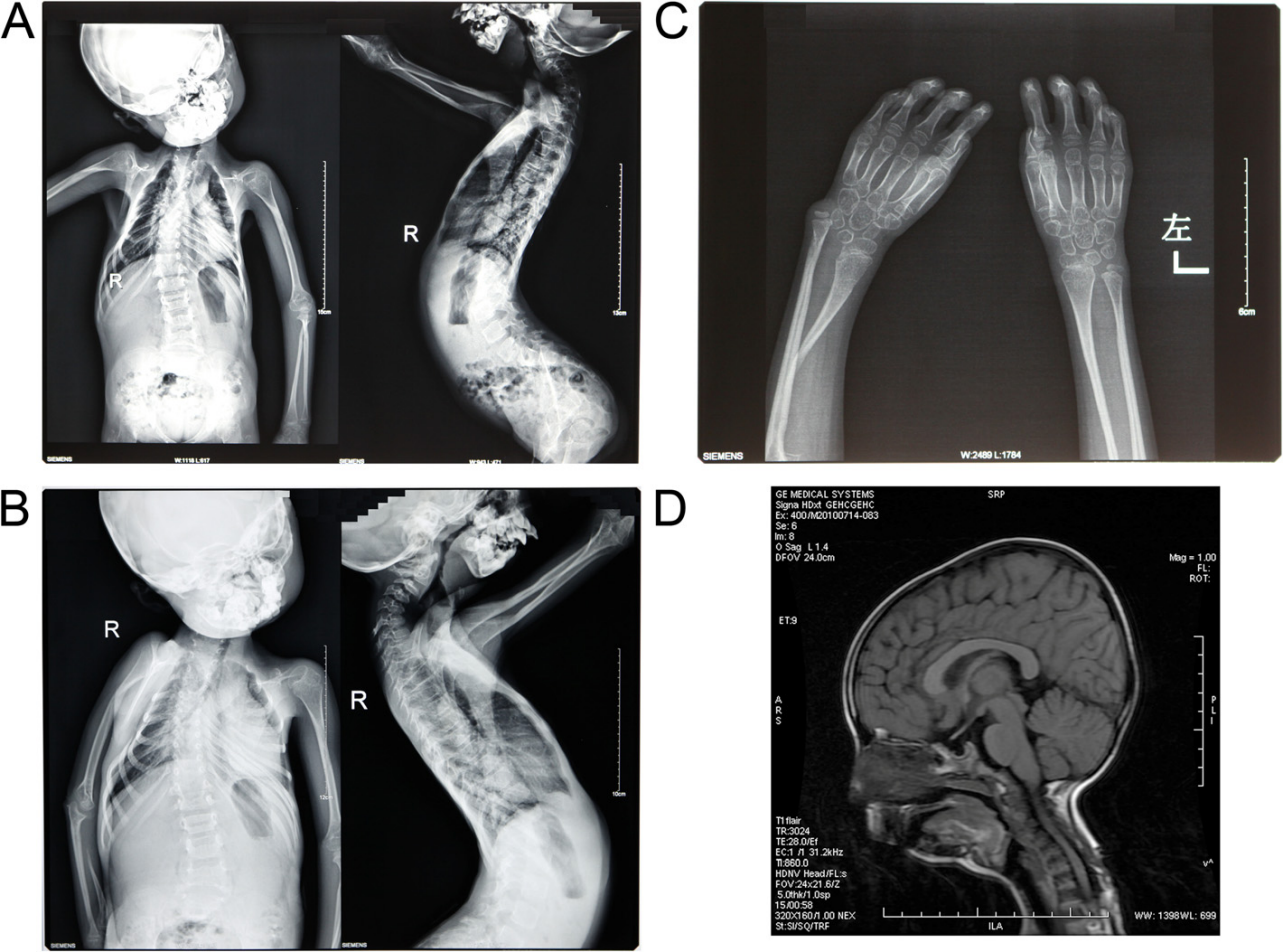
Radiography and magnetic resonance imaging features in case 1. (A,B) The elder sibling showed generalized and marked osteoporosis with relative expansion of the metaphyseal areas of the bones, completely absent clavicles, partial osteolysis of the bilateral posterior segments of the first to fourth ribs, absence of the costal head of the right 12th rib; severescoliotic deformities, complete osteolysis and resorption of the superior fragment of both radii, a dislocation of the inferior segment of the right ulnoradial joint, and an old, healed fracture of the interior segment of the right ulna. (C) Fixed flexion deformities of the phalanges in the elder sibling with absence of the distal phalanges of the second to fifth fingers. (D) Magnetic resonance imaging features of the elder sibling showing a small pituitary gland with a concavity of the superior border (with a height of about 1mm).
